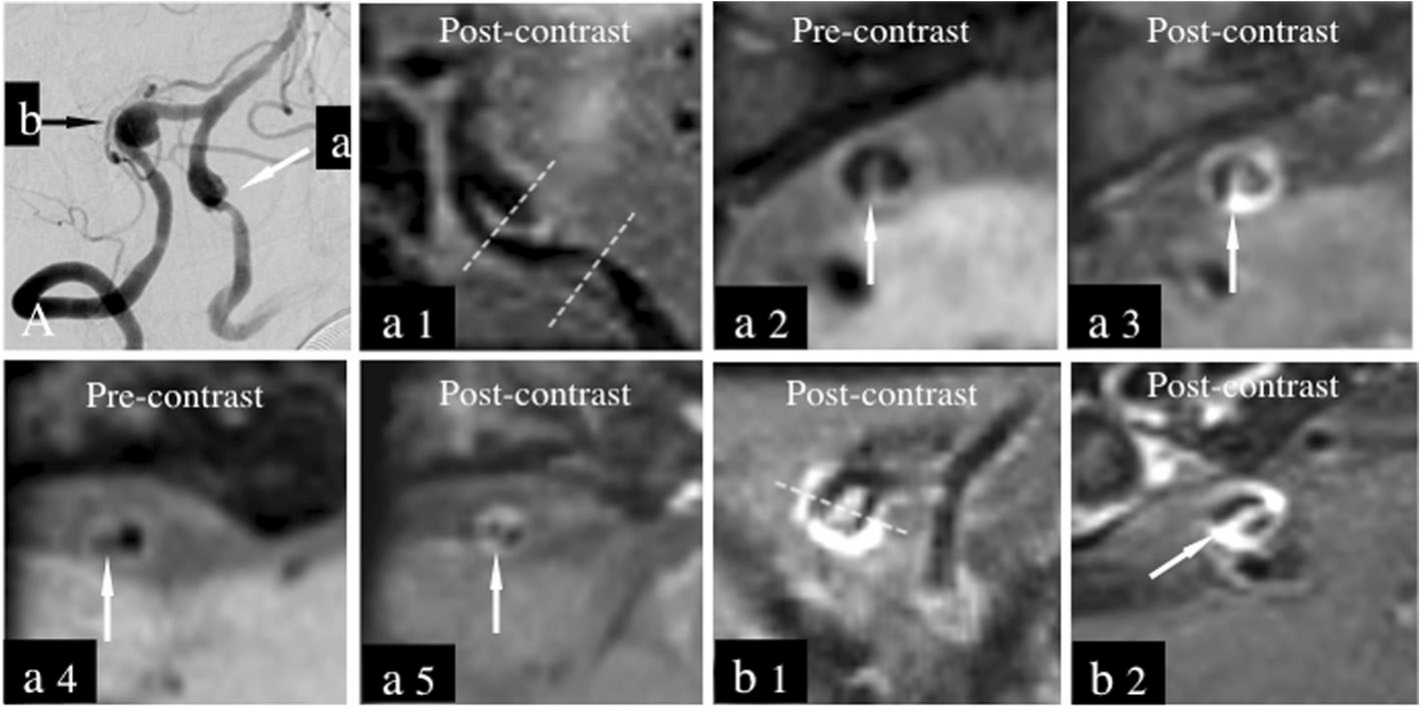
Patient 2. A 51-year-old man complained of dizziness and extremities weakness. Initial MRI was performed 11 days after symptom onset. Catheter angiography showed a lesion with a pear-and-string appearance (a, white arrow [a]) in the left intracranial vertebral artery and a lesion with aneurysmal dilation appearance (a, black arrow [b]) in the right side. VWI can be used for detecting the dissecting signs of both lesions simultaneously (a1-a5 for left side, b1-b2 for right side). Long-axis view of the lesion of left side revealed intimal flap and double lumen signs (a1, spotty line) on both the dilation and stenosis segments. Pre-contrast (a2, arrow) and post-contrast (a3, arrow) VWI showed intimal flap conspicuously on the dilation segment of left side (reconstructed at spotty line showed in a1). On the stenosis segment, intimal flap and double lumen was not detected on pre-contrast image (only eccentric wall thickness, a4, arrow) but on post-contrast image (a5, arrow). Long-axis view (b1, spotty line) and short-axis view (b2, arrow) of the lesion of right side revealed intimal flap and double lumen signs obviously
